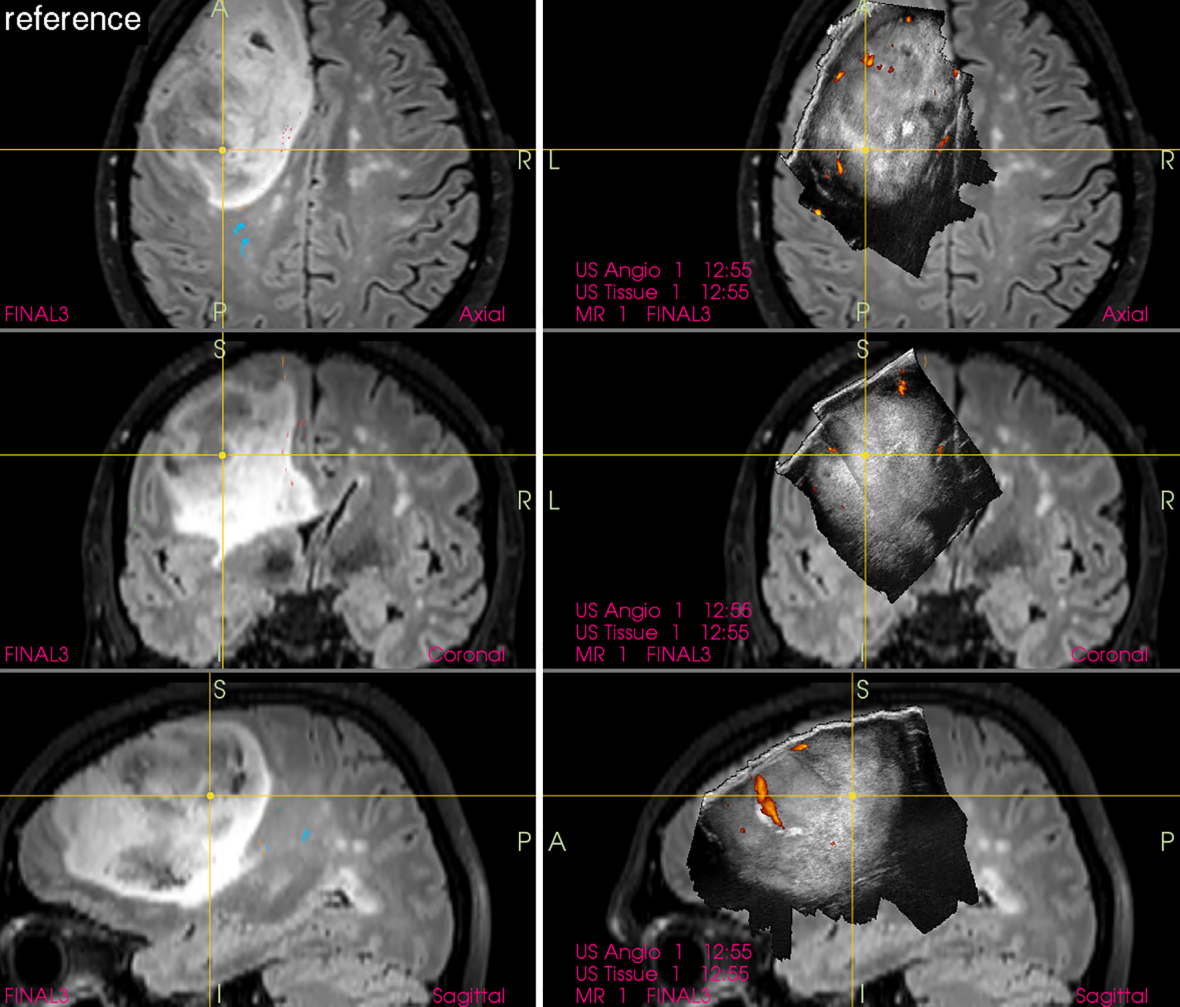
Left-sided frontal grade II astrocytoma (Left column) preoperative navigation 3D FLAIR MRI sequence (3-Tesla MRI scanner) (Right column) pre-resectional 3D IOUS image fused with navigation FLAIR MRI sequence. Note visualization of the entire tumor on 3D IOUS image despite its large size. 3D, three-dimensional; FLAIR, fluid attenuated inversion recovery; MRI, magnetic resonance imaging; IOUS, intraoperative ultrasound.
